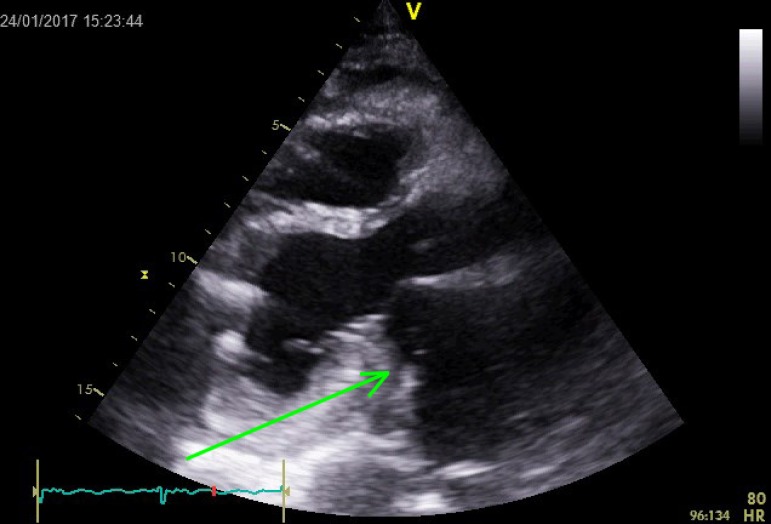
Follow-up echocardiogram showing residual thickening of the left atrial
